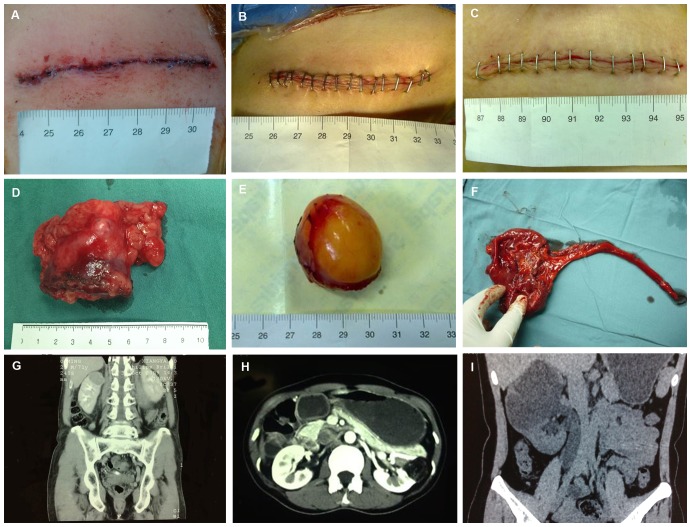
The supra-12th rib mini-flank approach for managing renal lesions. Renal clear cell carcinoma in the upper pole of the right side (A, D, G): The length of incision after MI-OPN (A); The length of the lesion after MI-OPN (D); Coronal reconstruction demonstrates a renal mass in the upper pole of the right kidney (G). Renal angiomyolipoma in the left side (B, E, H): The length of incision after MI-OPN (B); The length of the lesion after MI-OPN (E); Axial computed tomography (CT) scan shows a mass in the left kidney with low-density dark areas (H). Duplex kidney in the right side (C, F, I): C The length of incision after MI-OPN (C); The length of the lesion after MI-OPN (F); Coronal reconstruction demonstrates the right duplex kidney (I).
